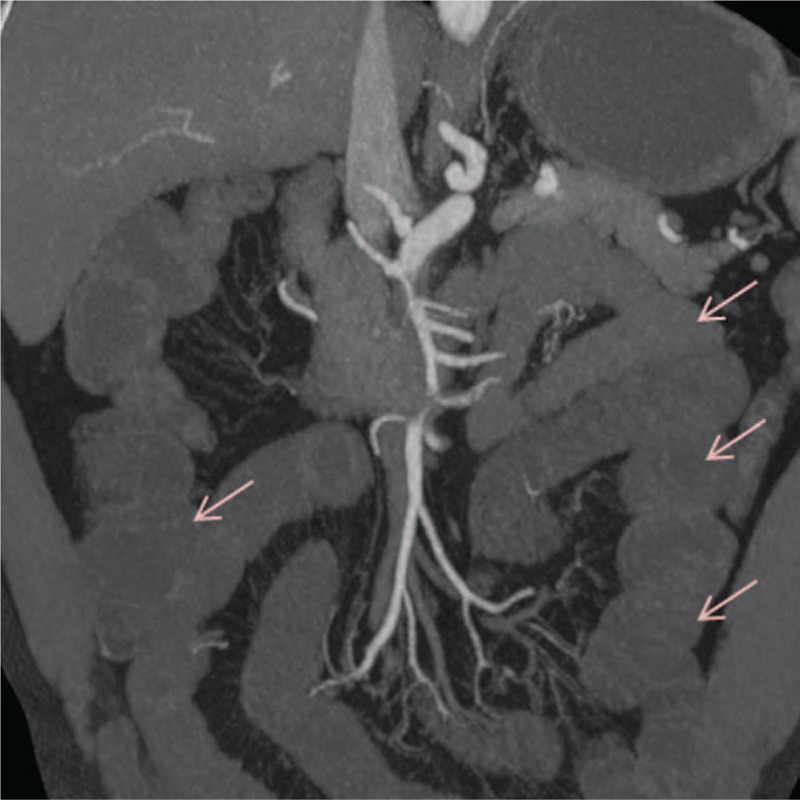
Coronary views of the small intestinal on enhanced computed tomography. Intestinal emphysema (arrow) was observed, suggesting intestinal necrosis.
